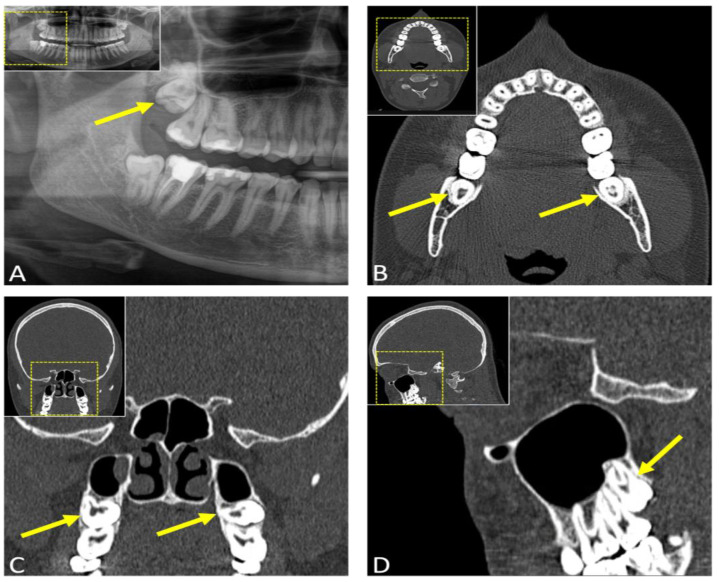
(A) Panoramic radiography (OPG) and (B–D) computed tomography (CT) of a 29-year-old female patient with an indication for third molar surgery. (A) The arrow points to the third molar in the upper first quadrant, while (B) axial, (C) coronal, and (D) sagittal CT reconstructions depict the upper third molars.
